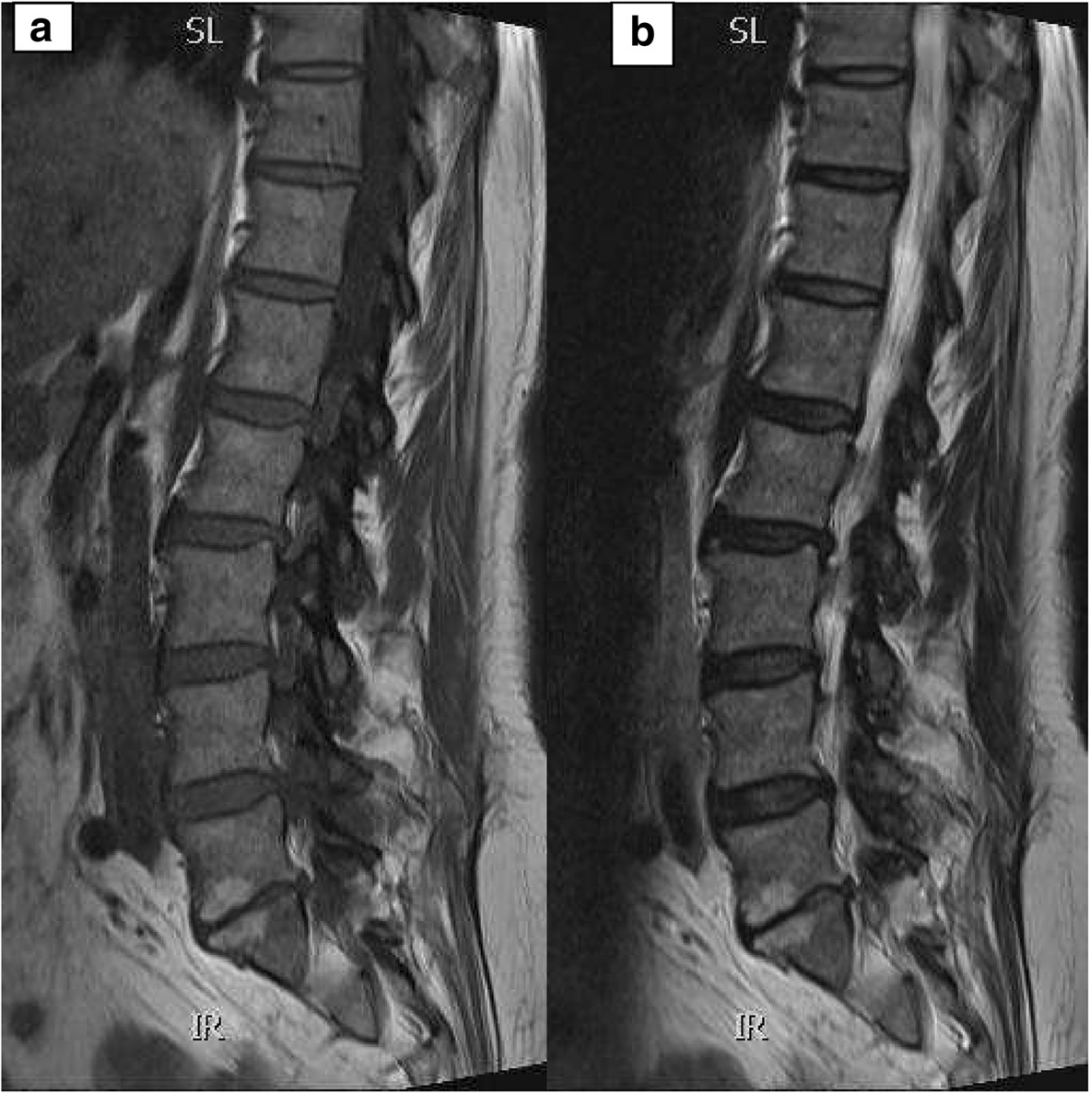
a and b Modic type II changes. T1 and T2 weighted sagittal MR images of a patient demonstrating high-signal intensity adjacent to the L5-S1 disc confirms MC type II marrow replacement. The L5-SI disc space is also narrowed
